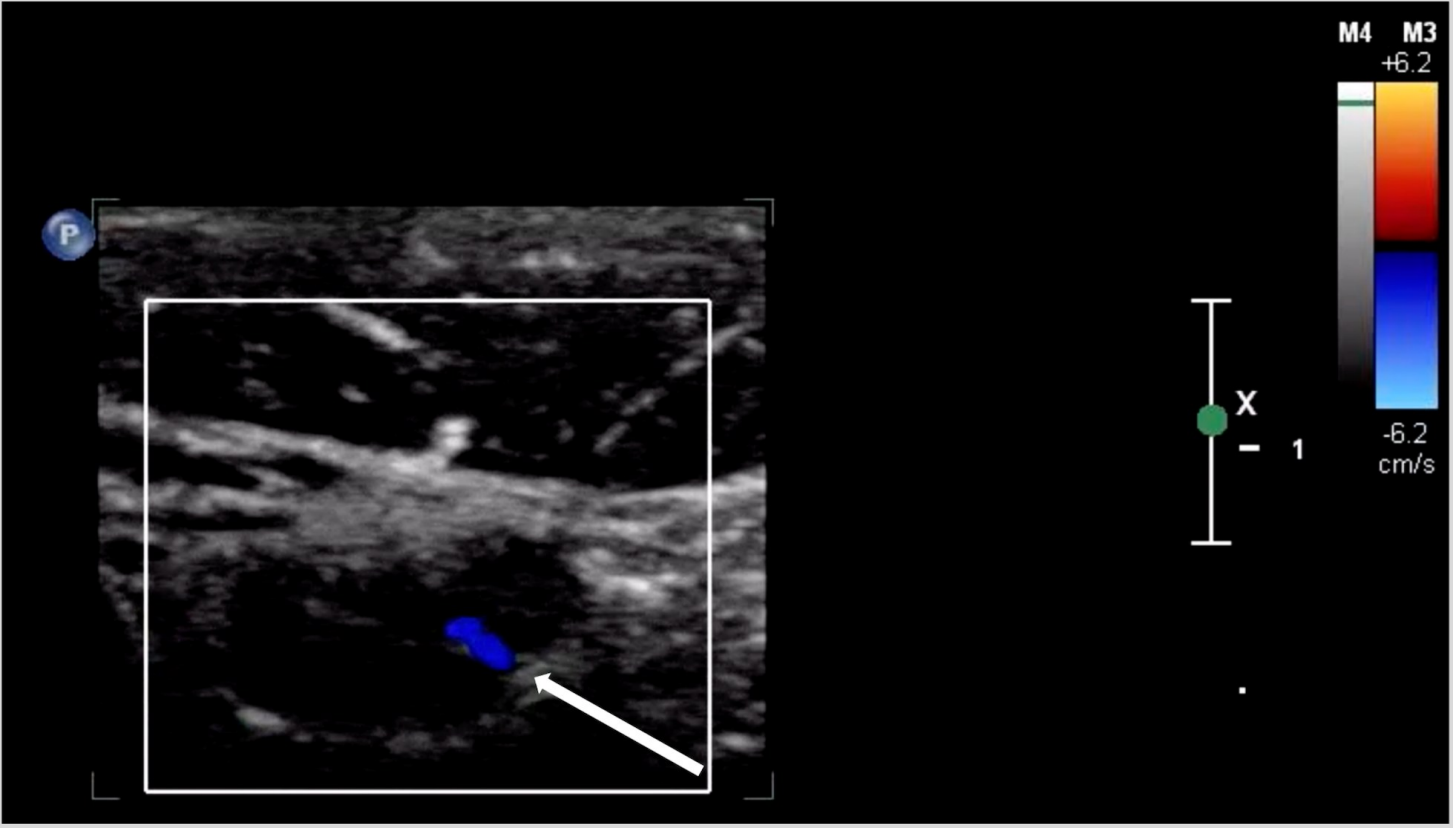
Point of care ultrasound (POCUS) with color Doppler applied to a normal lymph node (arrow indicating presence of hilum).
